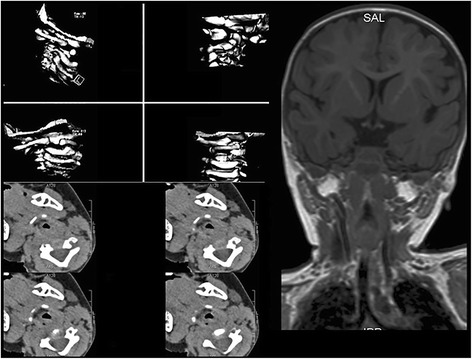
3D-CT reconstruction, axial and anterior-posterior NMR scan showing AAS and the typical head tilt
